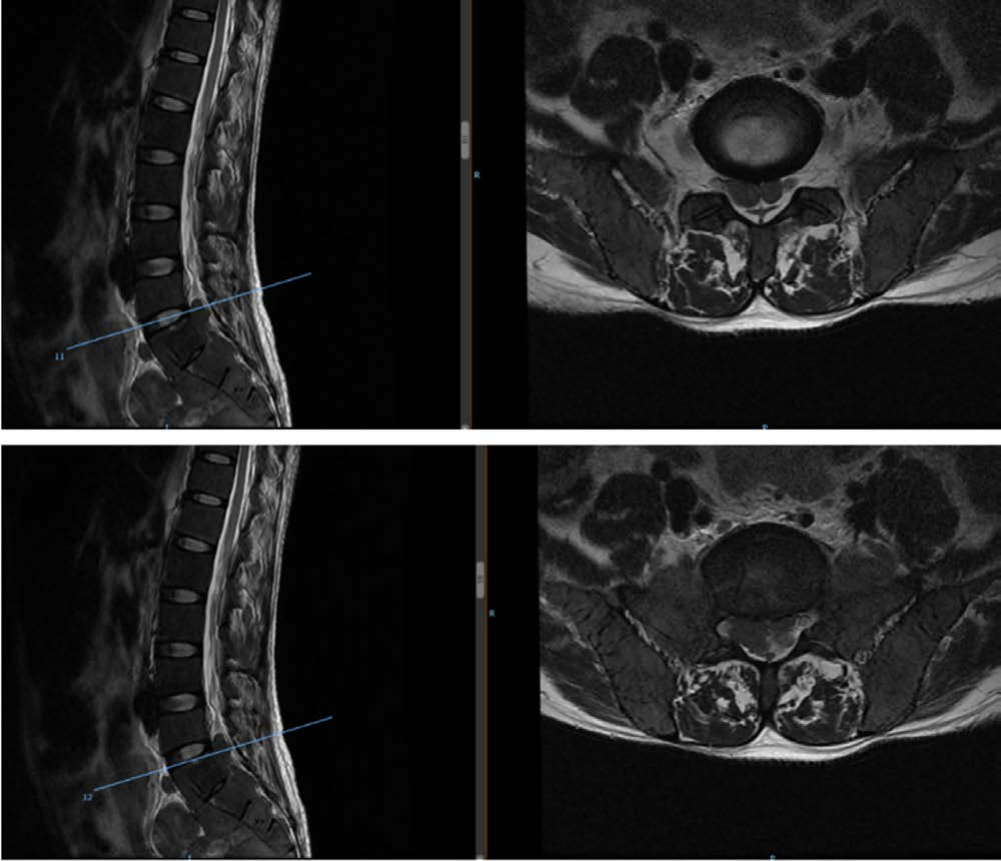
Preoperative magnetic resonance imaging (MRI) T2WI showing L5/S1 and S1/S2 levels secondary to spinal stenosis, and the spinal cord and cauda equina were inflexible.
